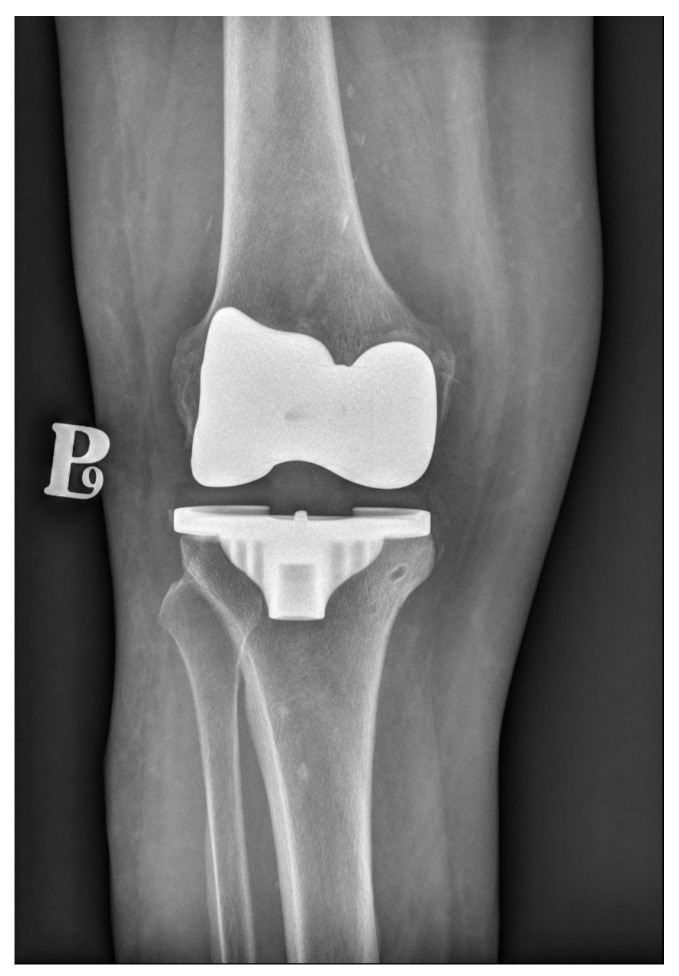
Conventional radiograph presenting cemented total knee replacement.
